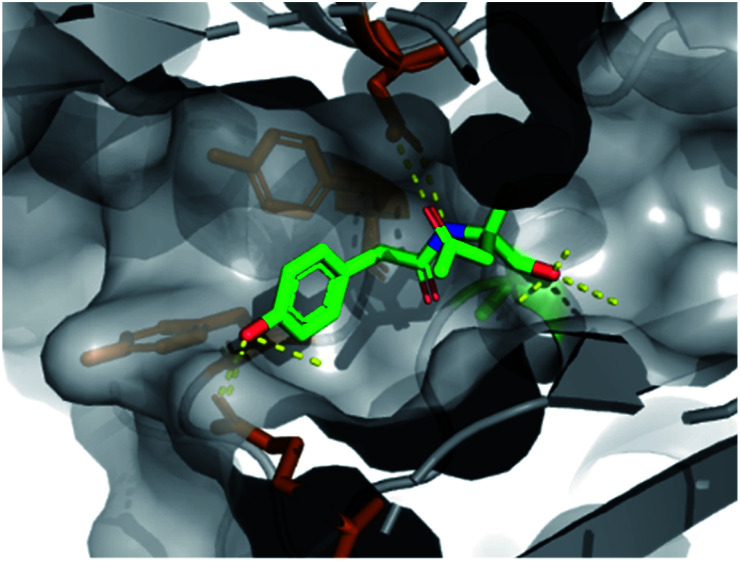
X-ray structure of 6 bound to PBP3 with conserved H-bonds highlighted.
